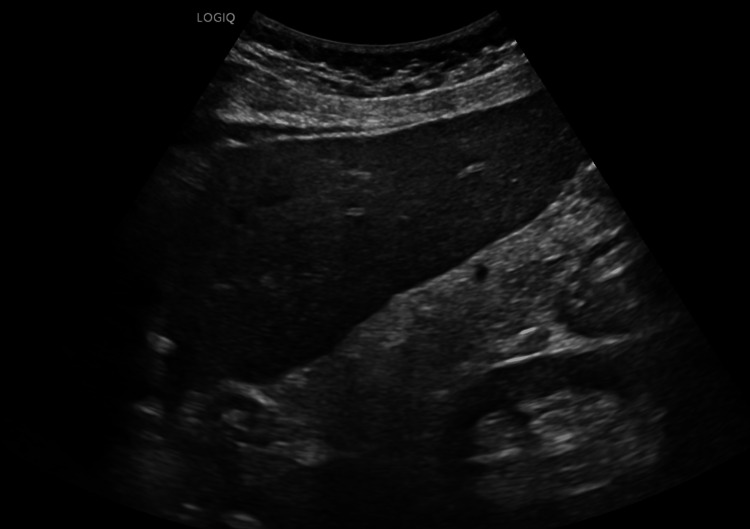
Two-Month Post-Discharge Follow-Up Liver Ultrasound. Two-month post-discharge follow-up ultrasound demonstrating a normal-appearing liver with no evidence of abscess. Mild focal fat deposition is seen along the porta hepatis.
